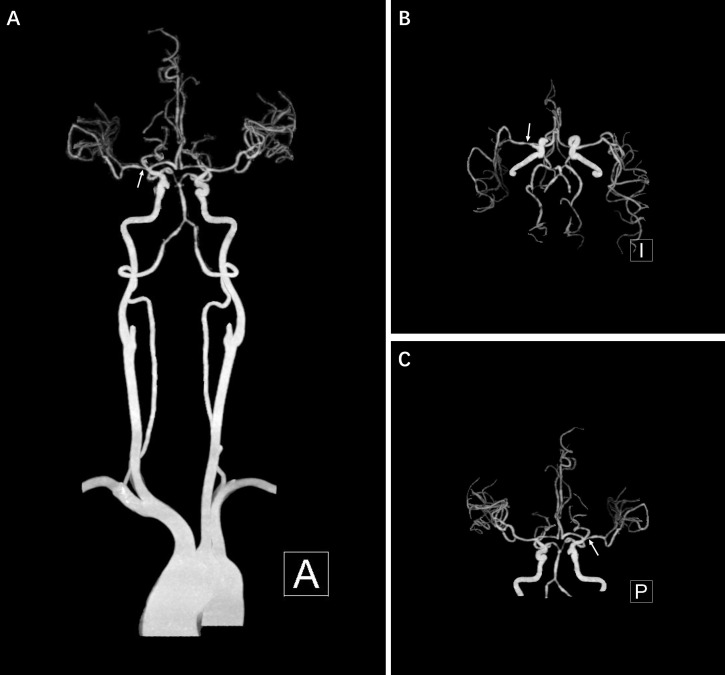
Head and neck CTA on the fourth day of admission. Severe stenosis in the M1-2 segment of the right middle cerebral artery.
